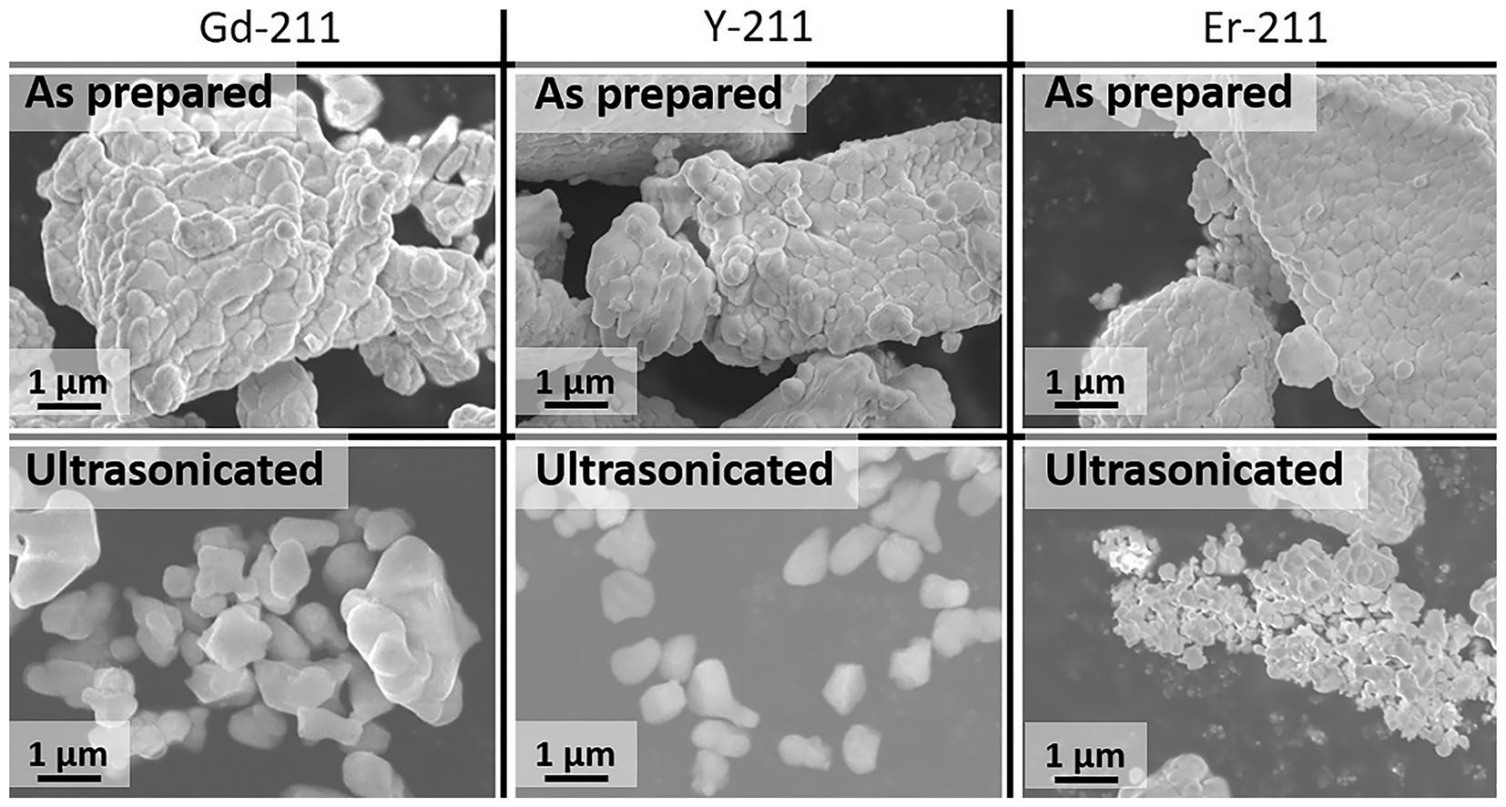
FE-SEM images of as prepared RE-211 powders (RE – Gd, Y, Er) via calcination before and after ultrasonication
